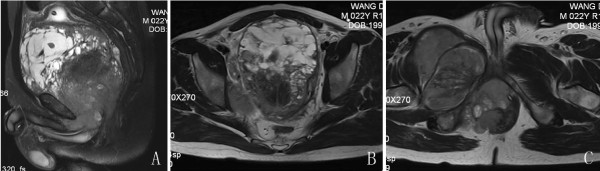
Enhanced pelvic magnetic resonance imaging. (A-C) Enhanced pelvic T2 weighted MR revealed a high signal mass originating in the prostatic fascia and an 8.5-cm mass was found in the right groin area.
